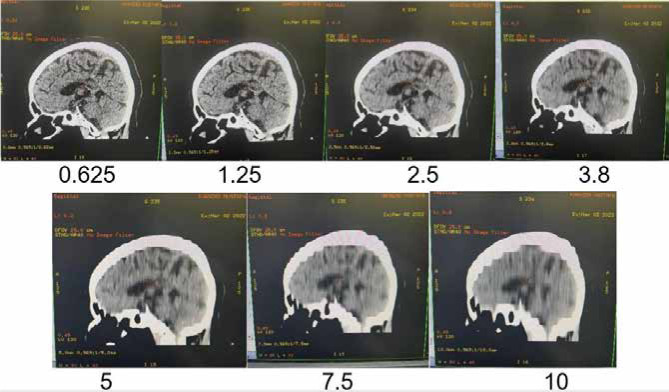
CT scan images with different thicknesses
